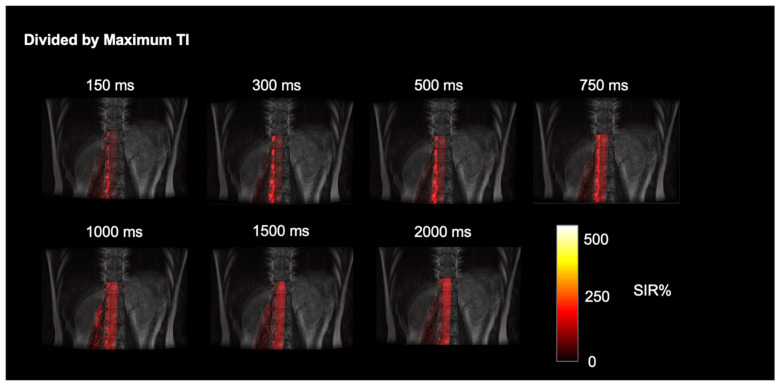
Time-resolved dynamic CSF outflow images fused with FLAIR images. SIR images at each TI, of subtracted images of tag-on and tag-off divided by maximum TI. The tagged CSF outflow from the nerve roots and egress over the psoas muscle is shown.
